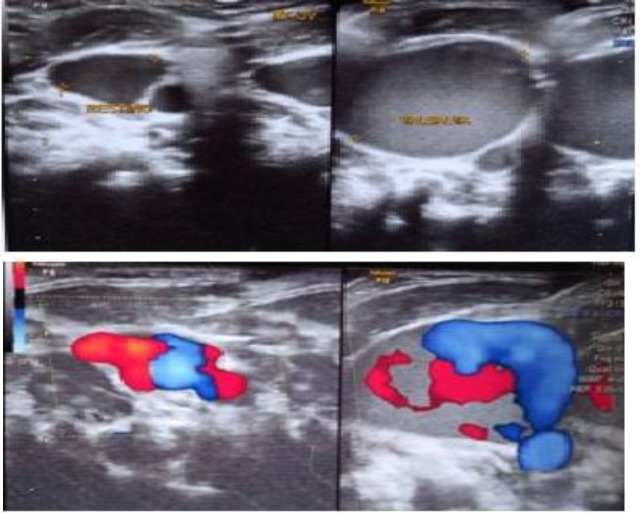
A- showing pre valsalva and post valsalva diameter of Right IJV on Ultrasound Neck.B- showing pre valsalva and post valsalva diameter of Right IJV on Color Doppler
